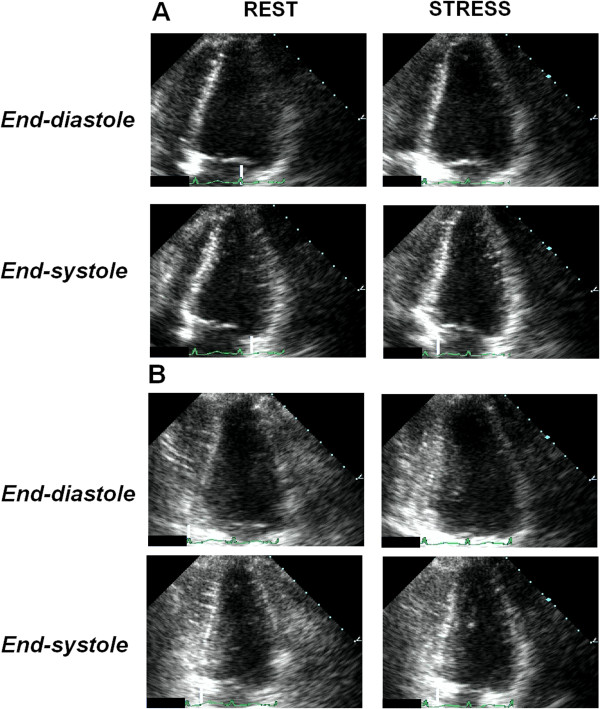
Donor #4 of Table 1. Transthoracic pharmacological stress echo test using dipyridamole (0.84 mg/kg in 6’) in a potential donor with prolonged (37 min) cardiac arrest at death [see Additional file 5]. The donor had normal response with normal regional wall motion during pharmacological stress echo. (panel A, 4-chamber views; panel B, 2-chamber views). The pressure/volume relation was positive and the individual was considered a suitable donor.
